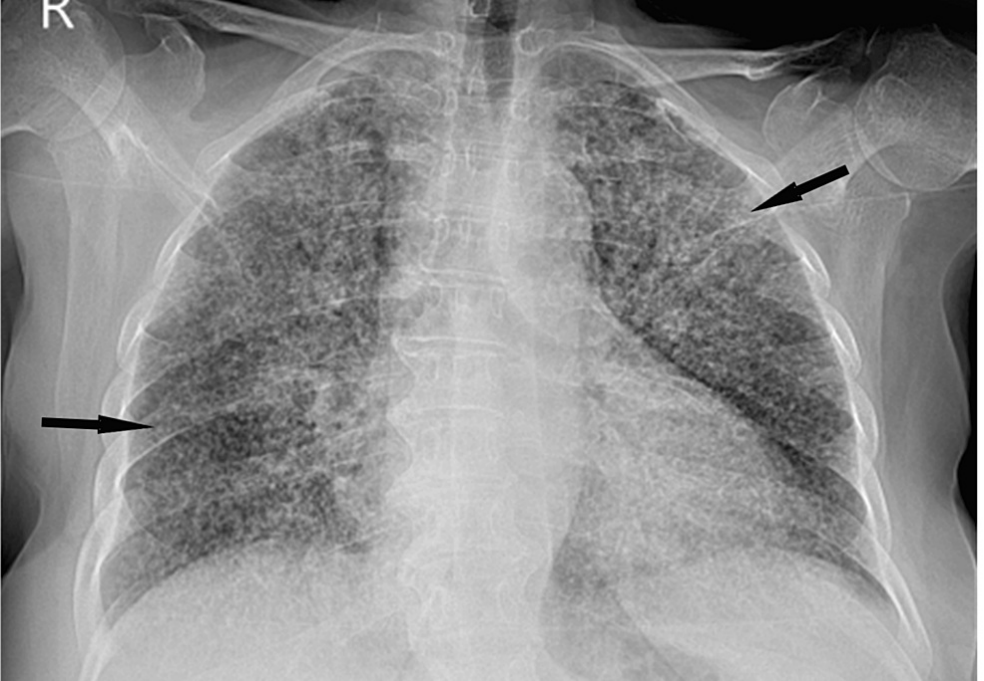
Chest X-ray on admission Arrows show diffusely distributed infiltrates with micronodular patterns.
